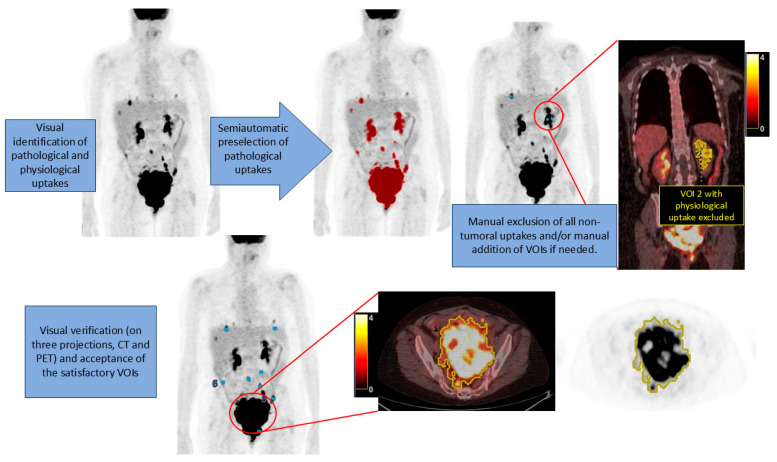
Reading and segmentation method for PET/CT images. VOI: volume of interest. Blue dots: locations of the VOI. Yellow line: VOI contour. Color bar indicates SUVmax values.
